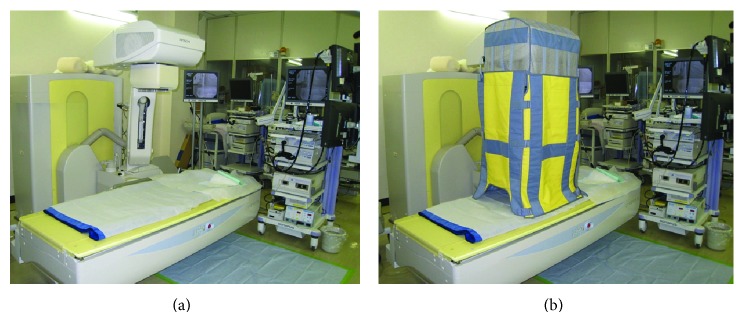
X-ray fluoroscopy generator and protective lead shield. (a) Without protective lead shield. (b) With protective lead shield.
